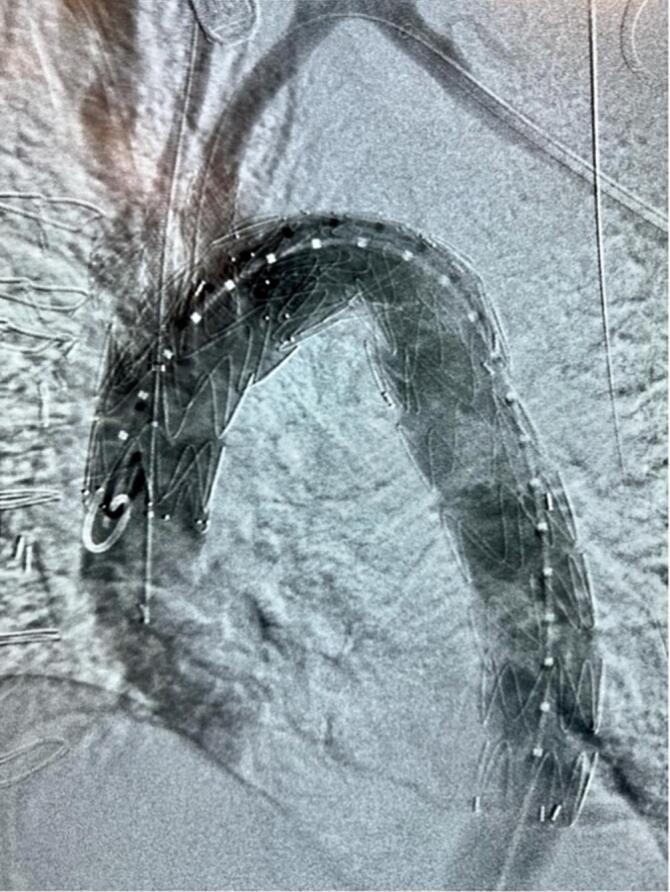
CT angiogram displaying blood flow following third stent placement.
